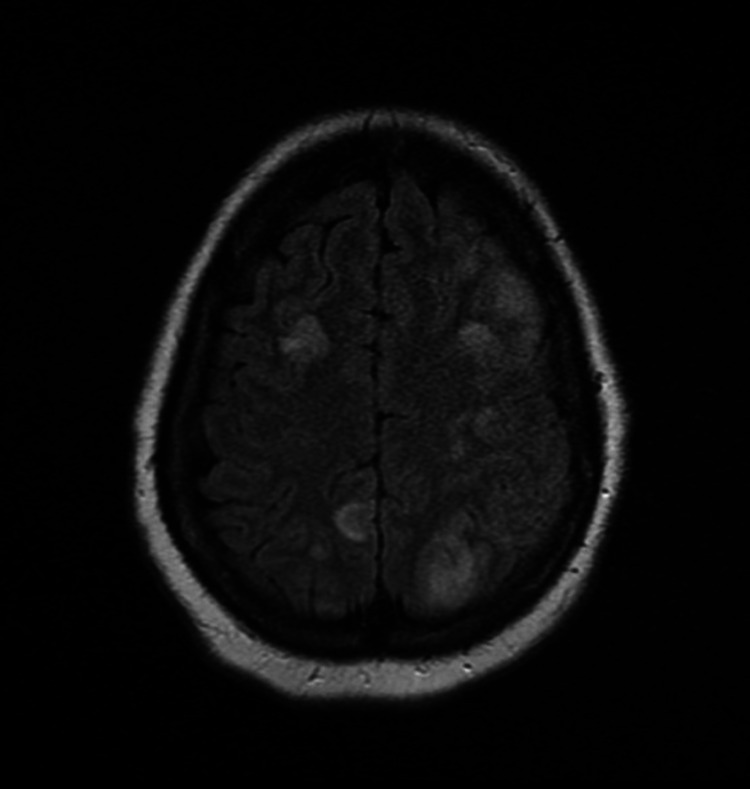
T2-weighted MRI axial imaging revealing multiple bilateral infarcts in the cerebral cortex
